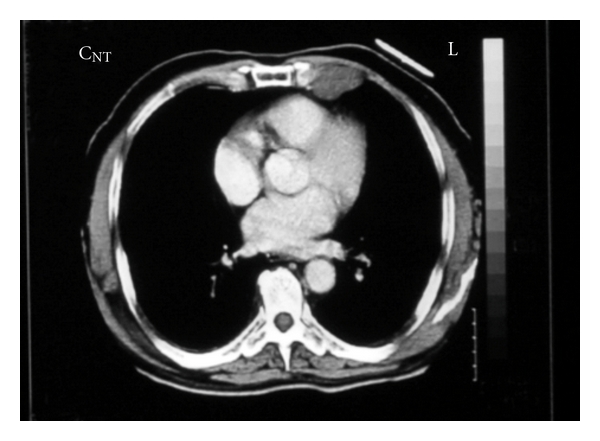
Computed tomography shows a homogeneous large cystic mass with a small solitary nodular area at the inferior margin.
